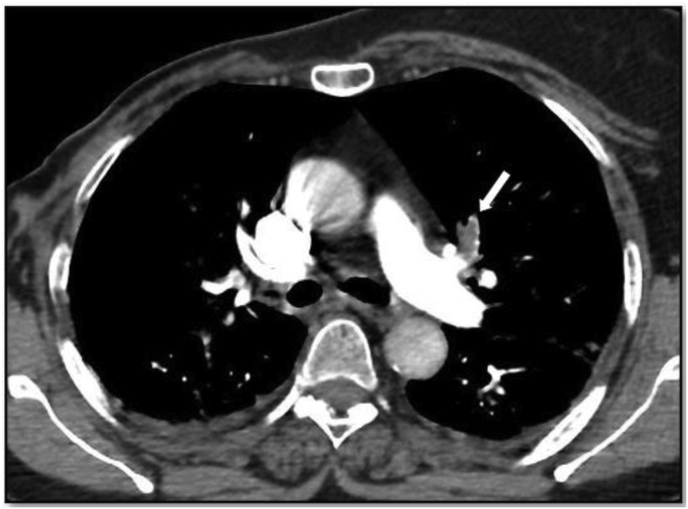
Axial sequence of a contrast-enhanced CT in mediastinal window showing a filling defect (arrow) in the left lobar pulmonary artery suggestive of pulmonary embolism.
